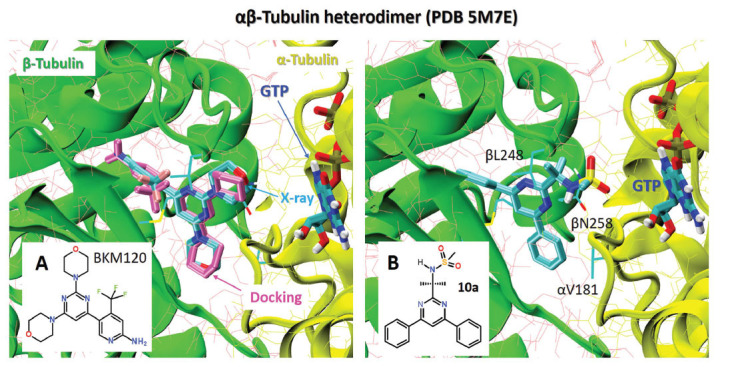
(A) Comparison of the best docking mode of BKM120 (magenta) with its X-ray structure (cyan) in αβ-tubulin heterodimer (PDB 5M7E). (B) The best docking mode of 10a in the active center of the tubulin receptor.
